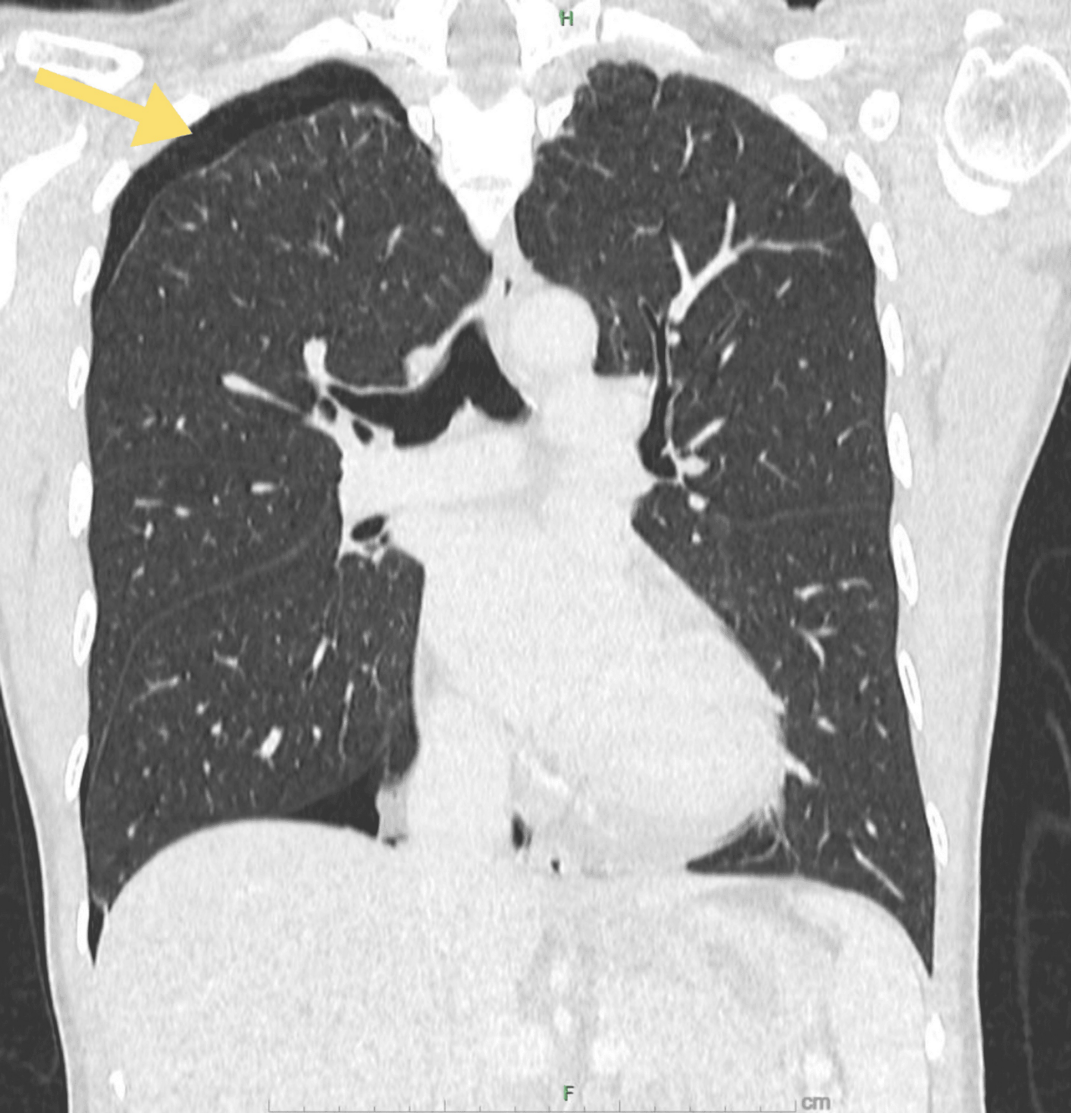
CT of the thorax (coronal view). The image shows a right apical pneumothorax (shown by the yellow arrow).
